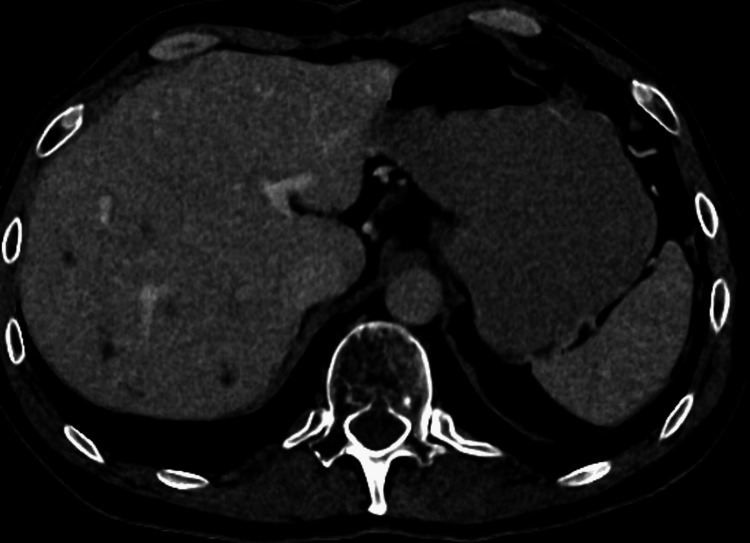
CT scan showing liver metastases prior to commencing alectinib CT: computed tomography
